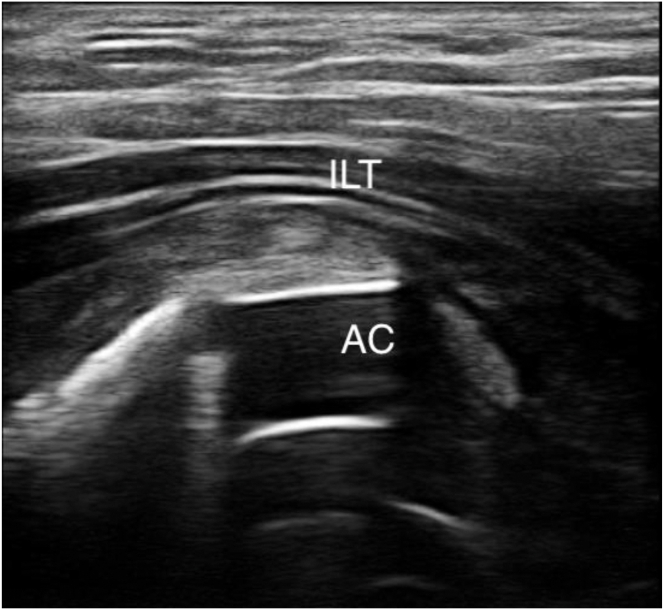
Ultrasonographic axial oblique view of iliopsoas tendon (ILT) with acetabular cup (AC) impingement.
